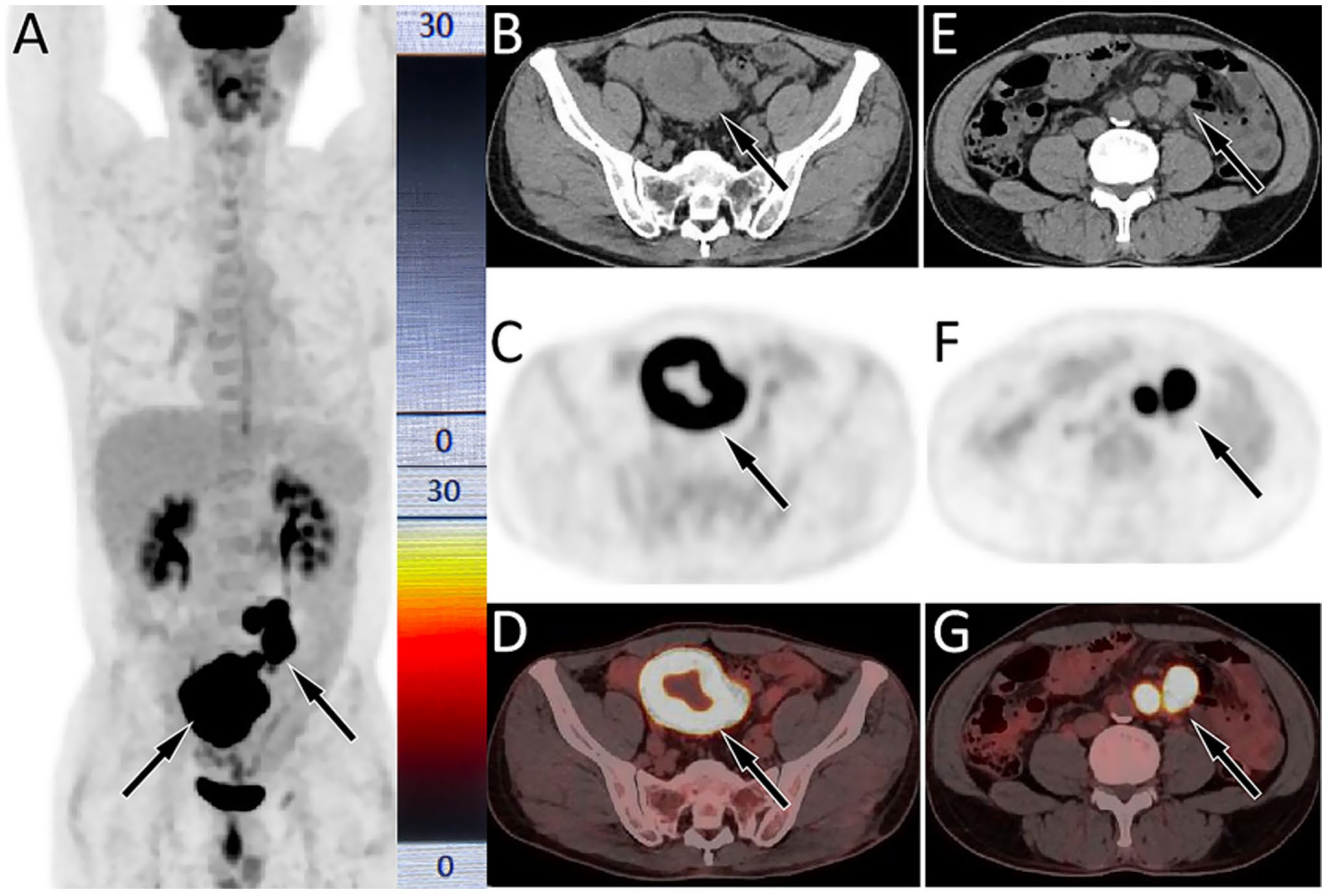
^18^F-FDG PET/CT imaging (injected activity is 303.4 MBq) on February 21, 2024; The MIP (A) showed multiple significantly increased ^18^F-FDG uptake in the middle and lower abdomen (arrows). Axial CT (B) showed uneven annular thickening of the small bowel near the ileocecal region (arrow). The corresponding lesion had obviously increased ^18^F-FDG uptake on axial PET (C, arrow) and PET/CT fusion (D, arrow), with a maximum standardized uptake value (SUVmax) of 22.1. (E) Axial CT also showed multiple enlarged lymph node adjacent to the abdominal aorta (arrows); which were significantly increased ^18^FDG uptake on PET (F) and PET/CT (G), with a SUVmax of 21.6.
